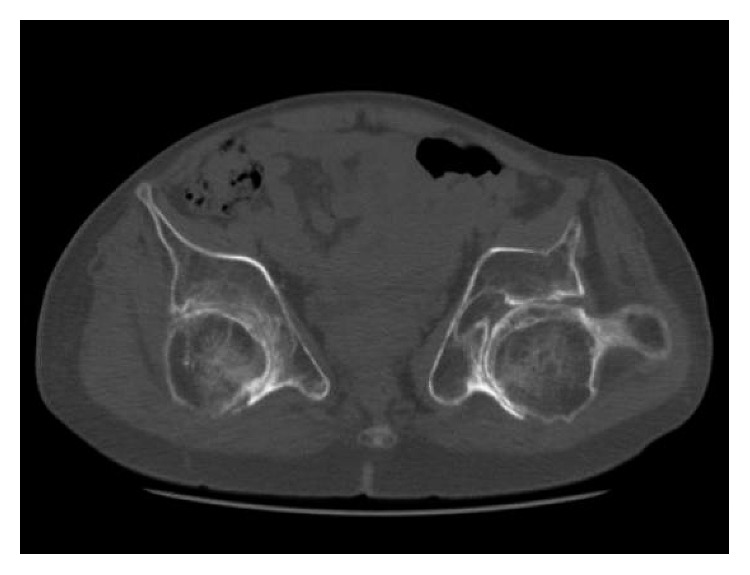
Axial CT image of the hip joints, demonstrating significant retroversion of the acetabulum.
